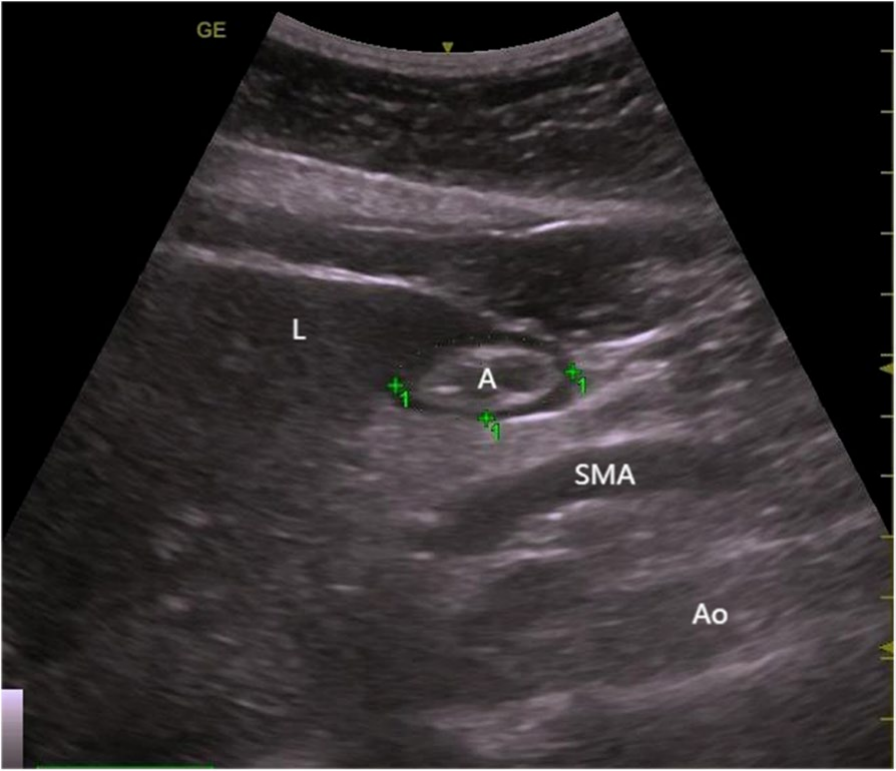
Ultrasonographic image of the gastric antrum. A: gastric antrum: L: liver; SMA: superior mesenteric artery; Ao: abdominal aorta
